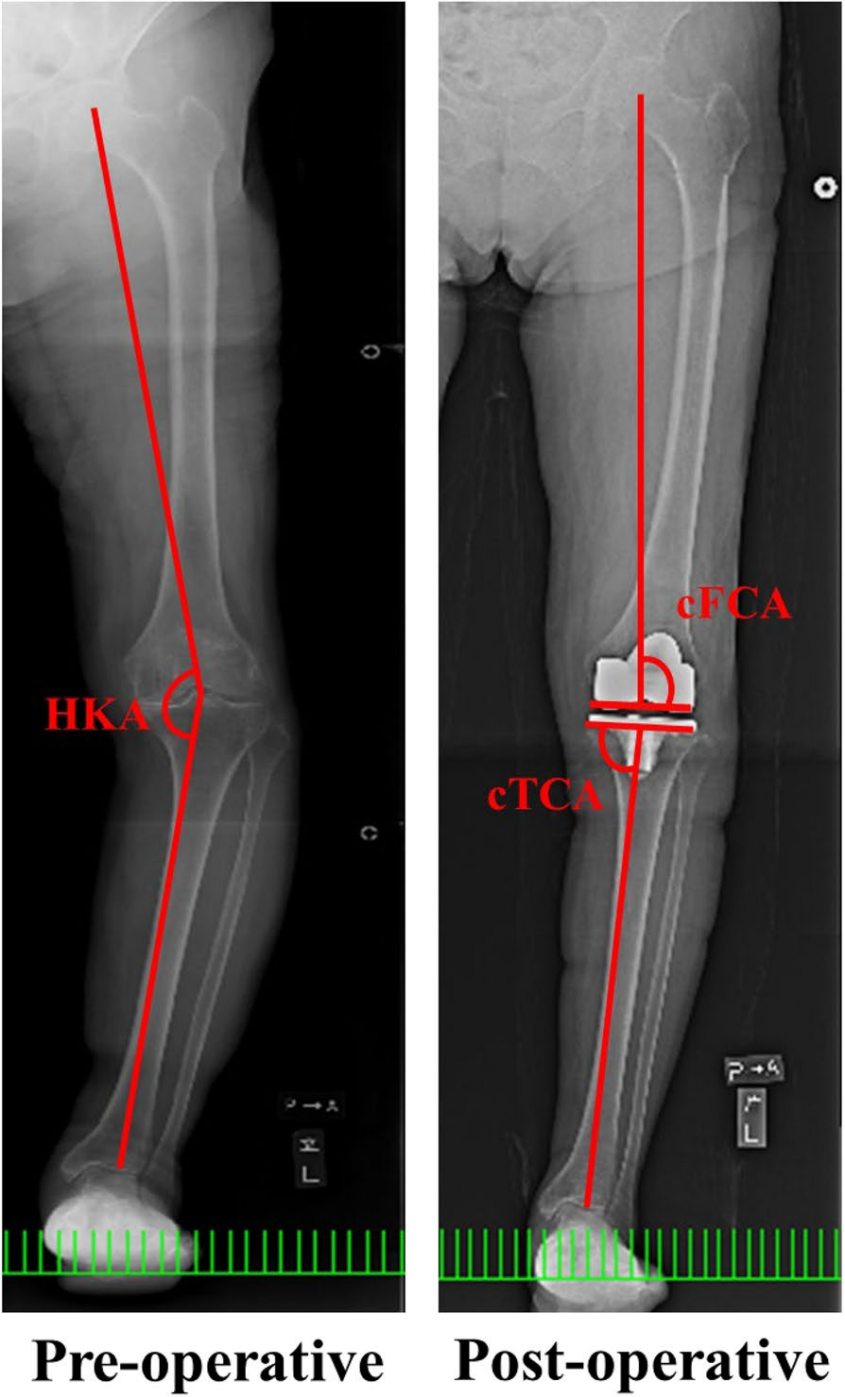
Pre- and postoperative X-rays of a patient who underwent restricted KA-TKA along with a radiographic evaluation. Hip‐knee‐ankle (HKA) angle is the internal angle formed by the femur and tibia’s mechanical lines. The coronal femoral component angle (cFCA) is defined as the external angle formed by the femoral mechanical axis and the tangent to the most distal part of the medial and lateral condyles of the femoral component on the coronal plane. The coronal tibial component angle (cTCA) is defined as the internal angle formed by the tibial mechanical axis and the tangent to the plateau of the tibial component on the coronal plane
